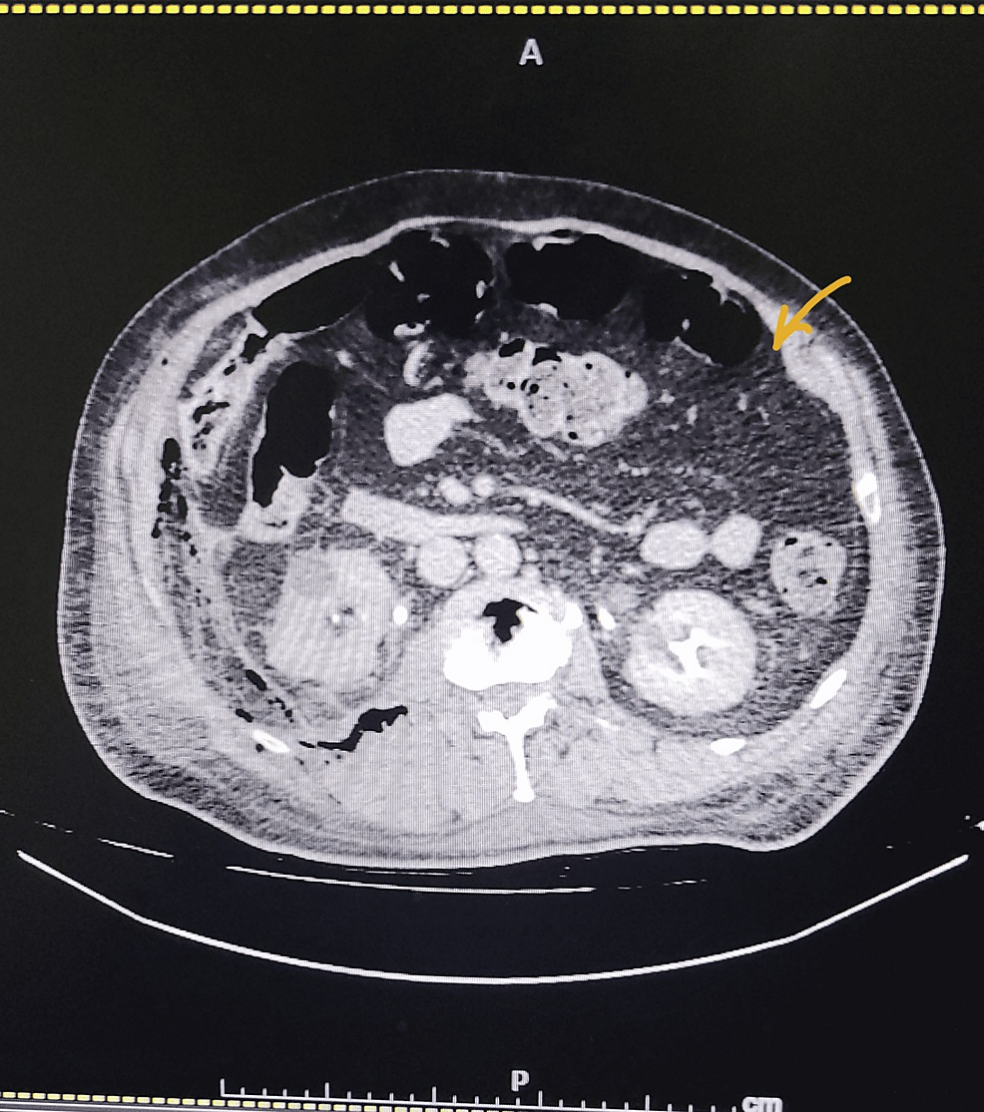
CT abdomen showing pneumoperitoneum CT: computed tomography
